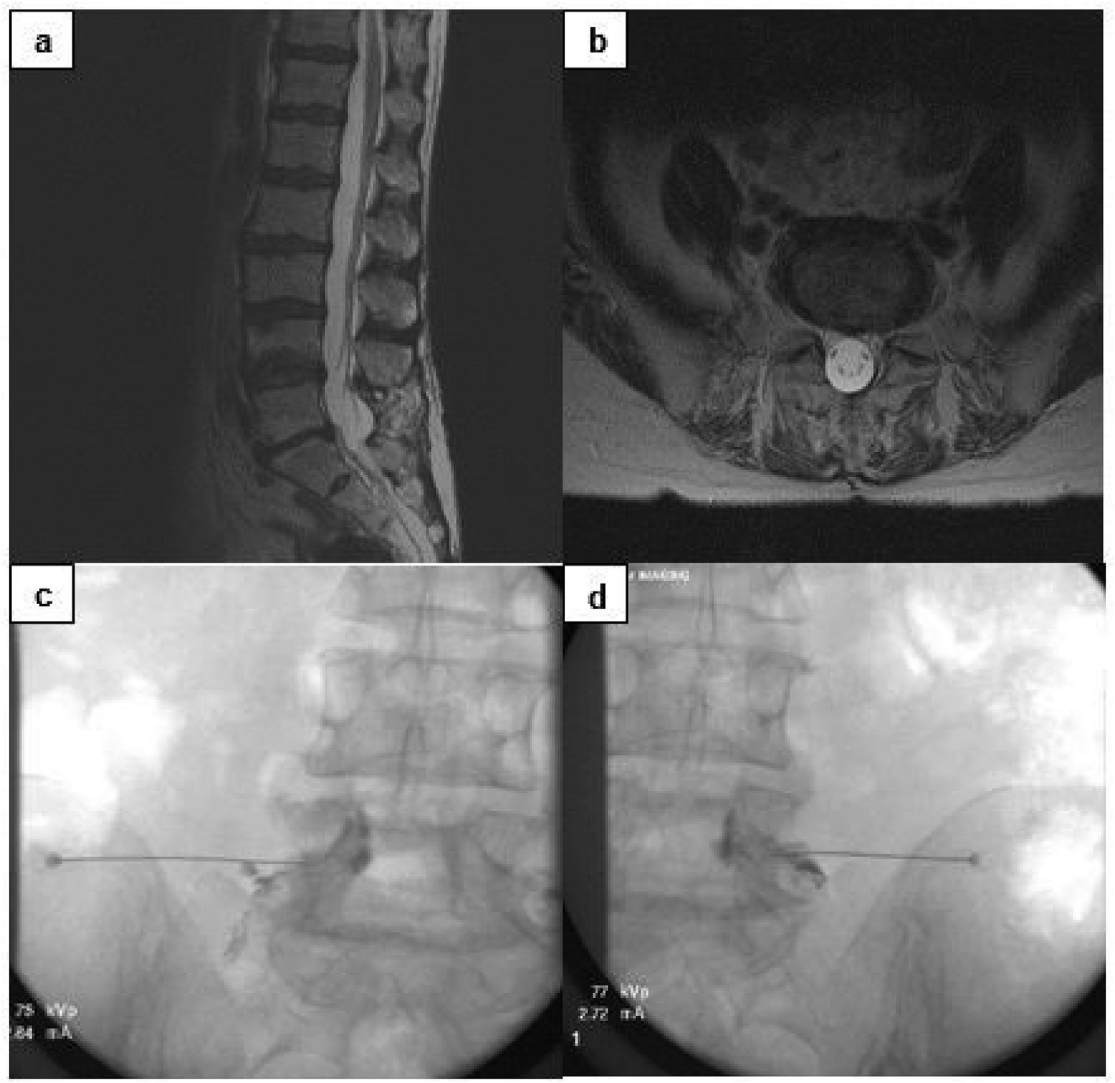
a) Sagittal T2-weighted MRI in a patient with previous laminectomy at L5-S1 b) Axial T2-weighted MRI at L5-S1 in same patient c) Fluoroscopic image showing Transforaminal epidural steroid injection at left L5-S1 d) Fluoroscopic image showing Transforaminal epidural steroid injection at right L5-S1 in same patient.
